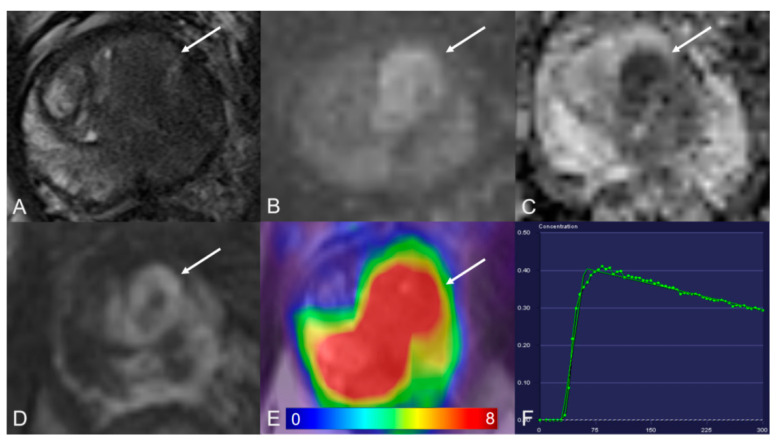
Transition zone with a PI-RADS 5 lesion. (A) Axial T2WI shows homogeneous hypointense. (B) DWI (b = 1000 s/mm^2^) shows a marked hyperintense signal above the background. (C) ADC map image presents a lesion with hypointense signal below the background. (D) Early dynamic contrast-enhanced image presents positive enhancement within the lesion. (E) [^68^Ga]Ga-PSMA-11 PET/MRI fusion image shows avid [^68^Ga]Ga-PSMA-11 uptake, with SUVmax of 29.2. (F) DCE-MRI time-intensity curve demonstrates a decline after initial up-slope enhancement. AT: 0.47 min; TTP: 0.50 min; W-in: 0.80; W-out: −0.03; PEI: 0.41; iAUC: 0.31.
